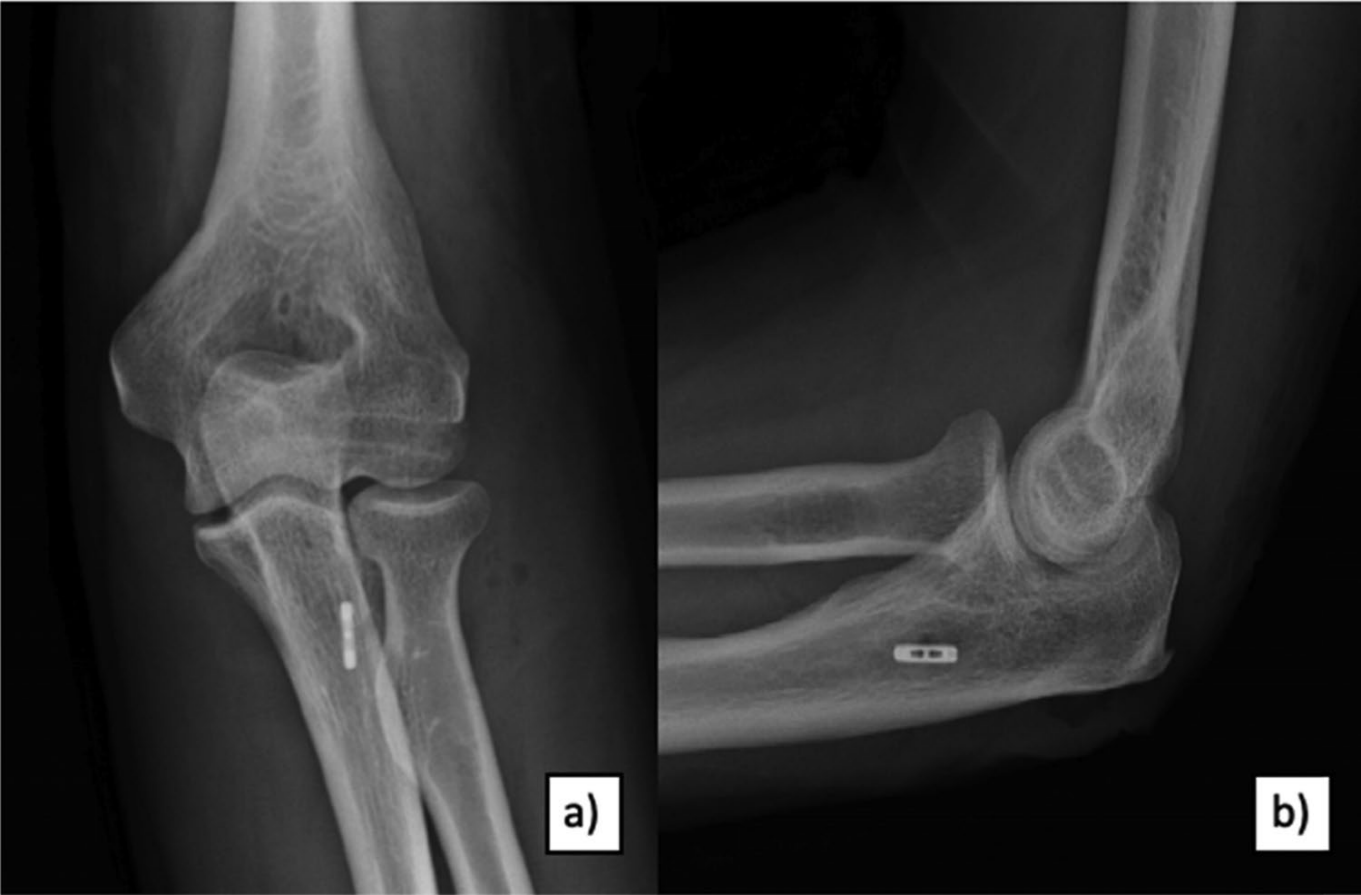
Postoperative X-rays a a.p. and b lateral after LUCL reconstruction using ipsilateral triceps graft: flip button fixation at the ulnar side and SwiveLock anchor (Arthrex Napels, FL, USA) fixation at the humeral condyle
